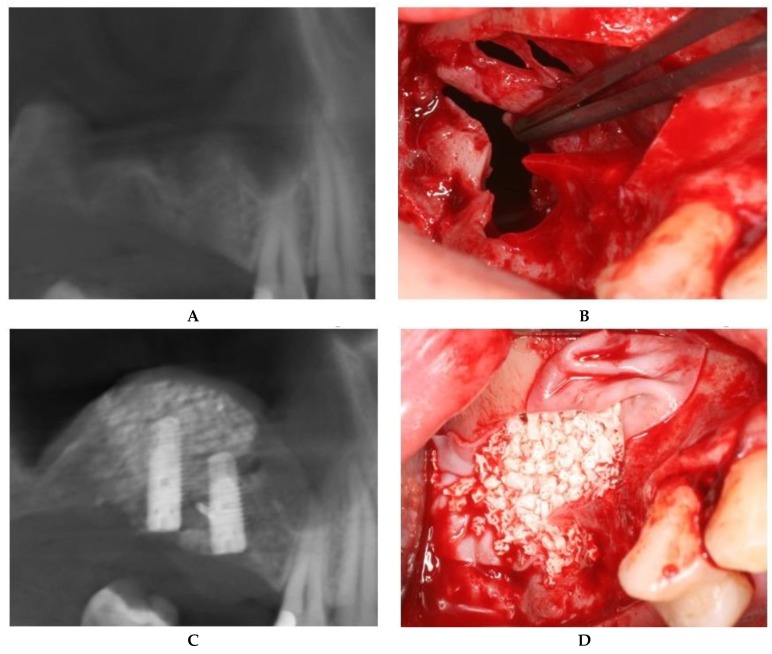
Schneiderian membrane sealing technique with collagen membrane coverage. (A) Preoperative CBCT image. (B) Large, multiple Schneiderian membrane perforations. (C) Bone graft placed in the collagen membrane “pouch”. (D) Postoperative CBCT after implant placement.
